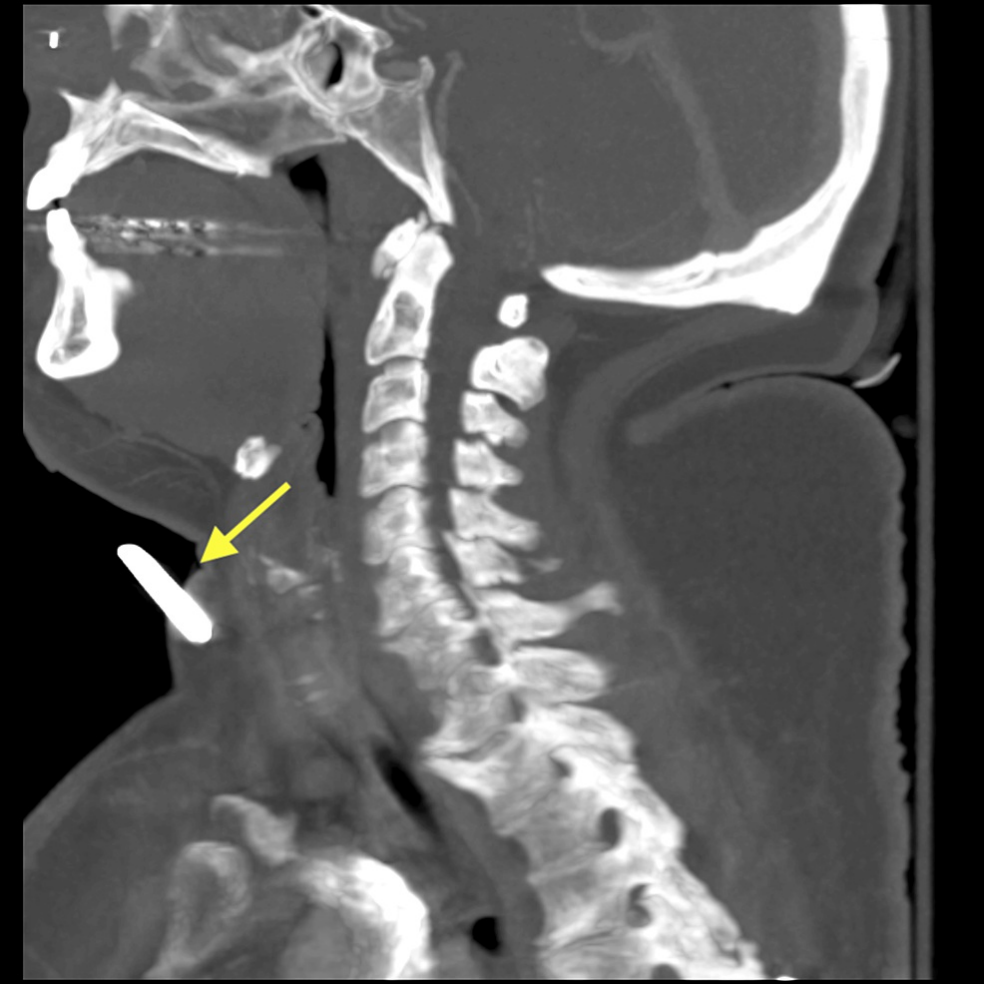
CTA of the neck showing a metallic object penetrating the anterior neck soft tissue CTA: computed tomography angiography
